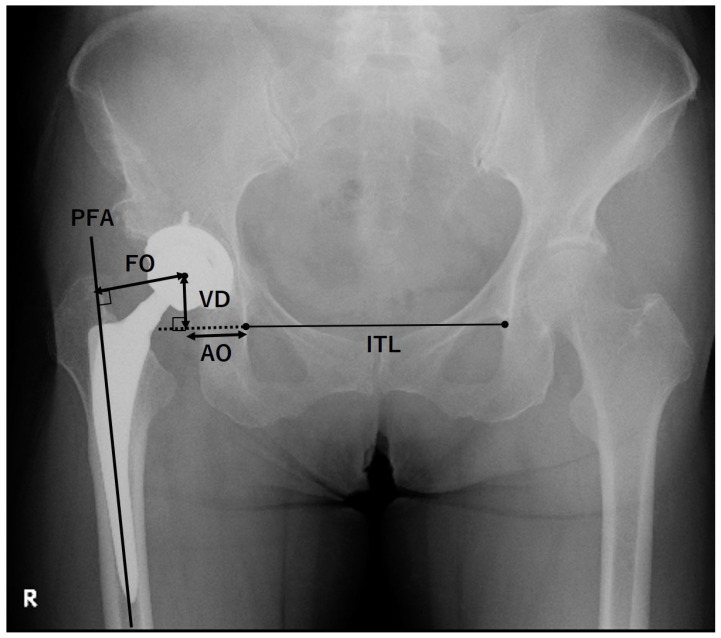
Radiographic indices measured on the anteroposterior radiographs. The interteardrop line (ITL) connects the inferior edges of both teardrops. The acetabular offset (AO) was measured as the horizontal distance from the inferior edge of the teardrop to the point where an extension of the ITL intersects with a perpendicular line drawn from the hip joint center. The vertical distance (VD) was measured as the distance from the hip joint center to the point where the extension of the ITL intersects with the perpendicular line from the hip joint center. The femoral offset (FO) was measured as the distance from the hip joint center to the intersection with the proximal femoral shaft axis (PFA). The global offset was measured as the sum of FO and AO.
